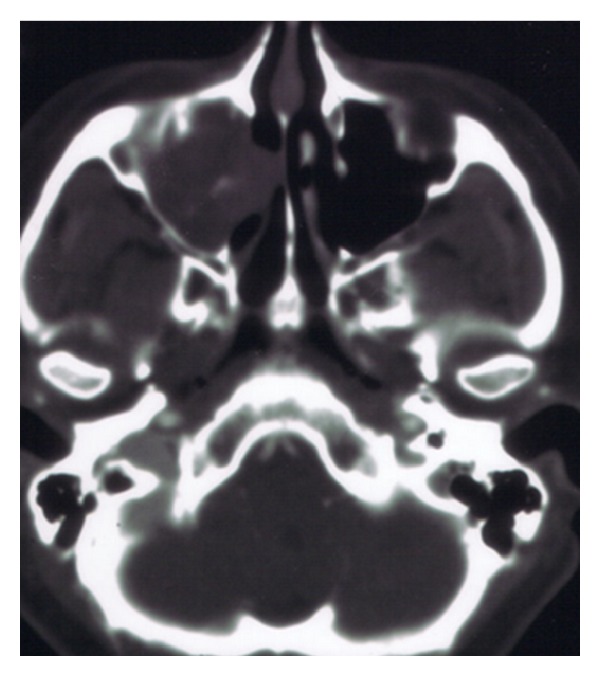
A 36-year-old female had a 2-month history of recurrent epistaxis. Initial coronal contrast-enhanced computed tomography scan of the paranasal sinuses revealed a 36 × 24 mm heterogeneous enhanced mass in the right maxillary sinus. The central region of the mass was strongly enhanced. Compression and thinning of the lateral wall of the right nasal cavity were observed (case III).
